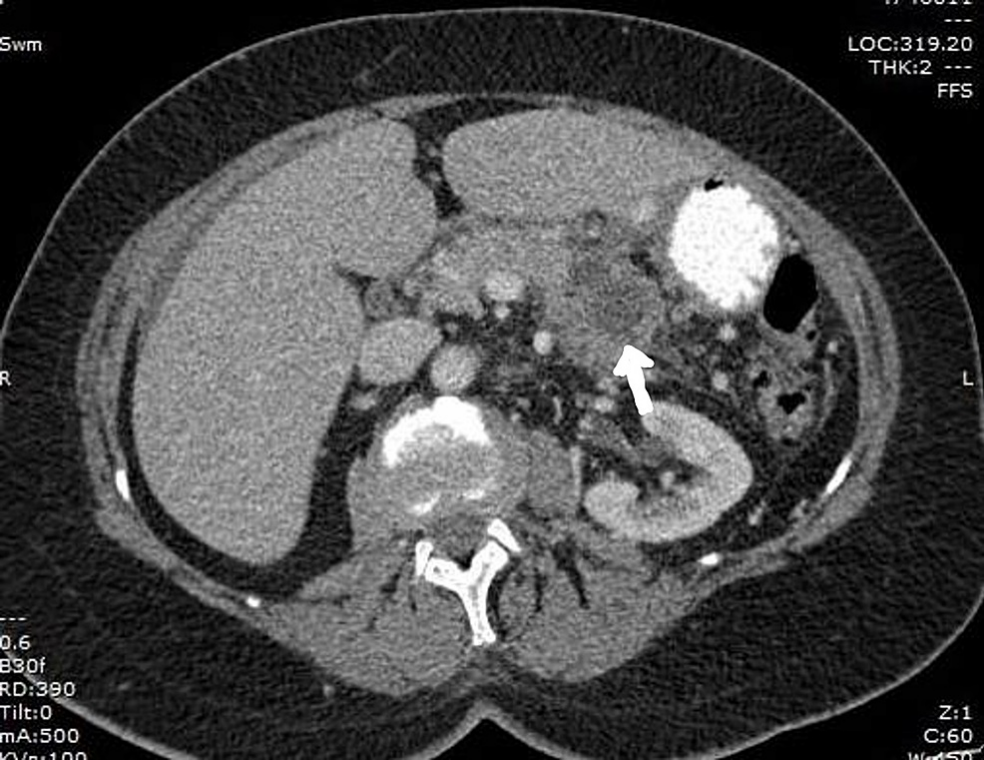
CT scan showing pancreatic tumor in the tail
